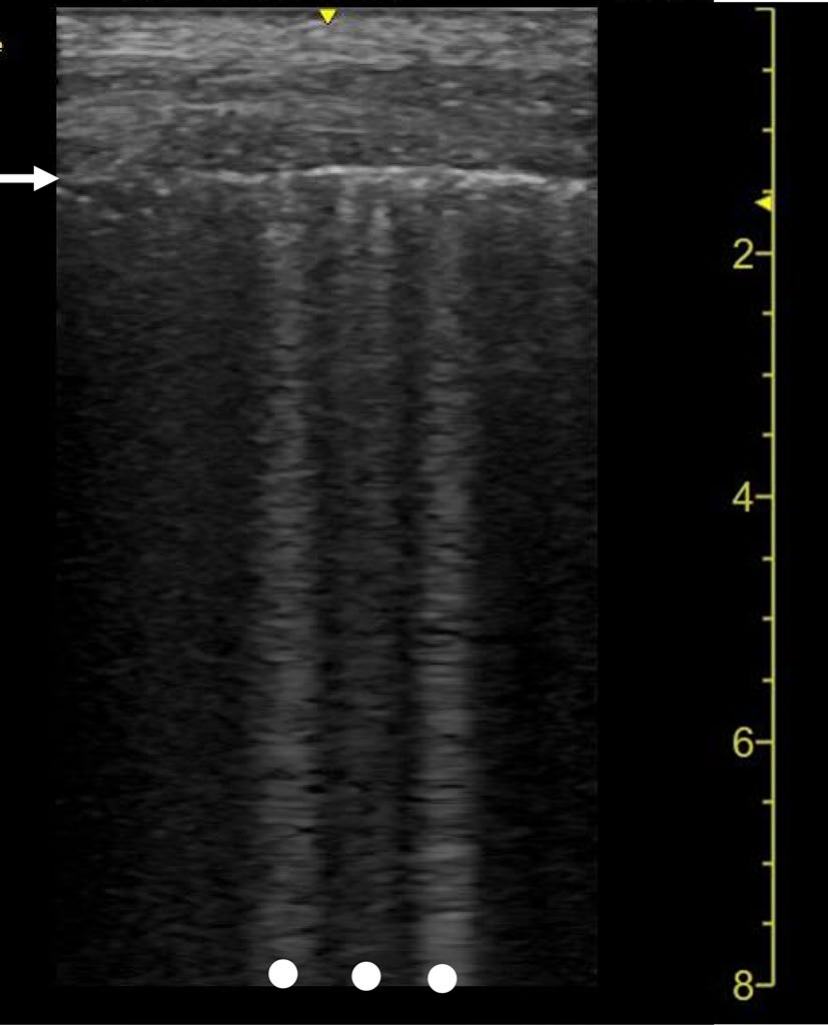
A lung ultrasound image of community-acquired pneumonia. Multiple B-lines (dots) are shown with irregularity of the pleural line (arrow)
